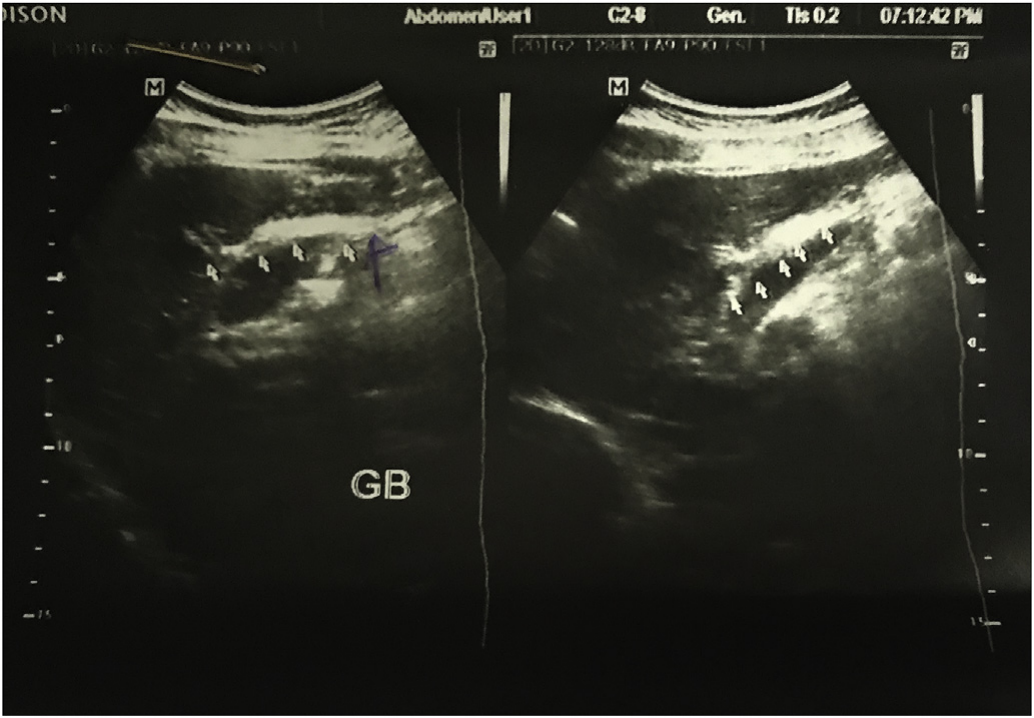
An ultrasound picture of the biliary tree showing a normal caliber of the common bile duct with suspicion of small and contracted gall bladder (the white arrows).
